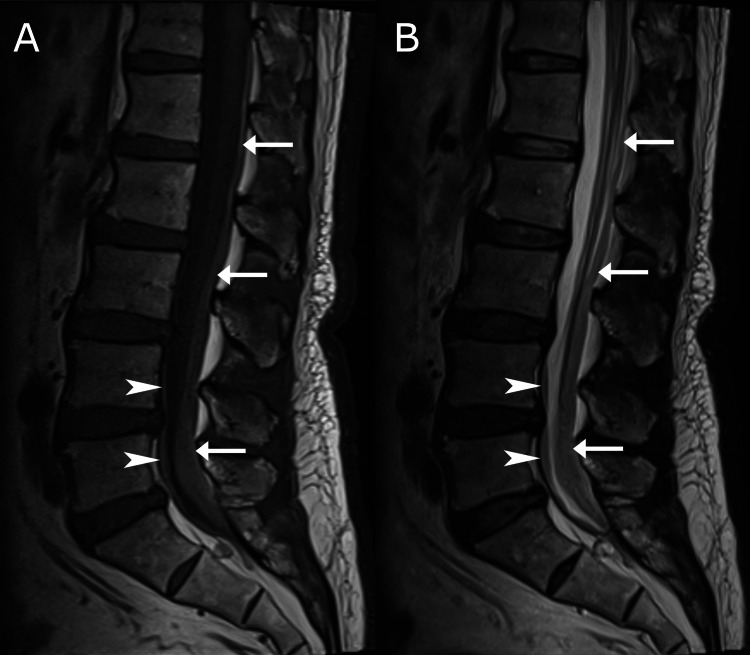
Sagittal lumbar MRI A T1-weighted (A) and T2-weighted (B) mildly hyperintense signal layer measuring up to 1 cm in anteroposterior diameter is seen along the anterior and posterior aspect of the spinal canal from T12/L1 to S1/2 levels, with tapered superior and inferior edges, appearing intradural in location (arrows), consistent with subdural hematoma. A thinner layer with a similar signal is seen at the anterior aspect of the spinal canal from L4 to S1/2 levels, measuring up to 0.4 cm in the anteroposterior diameter, also appearing intradural (arrowheads). MRI: magnetic resonance imaging
